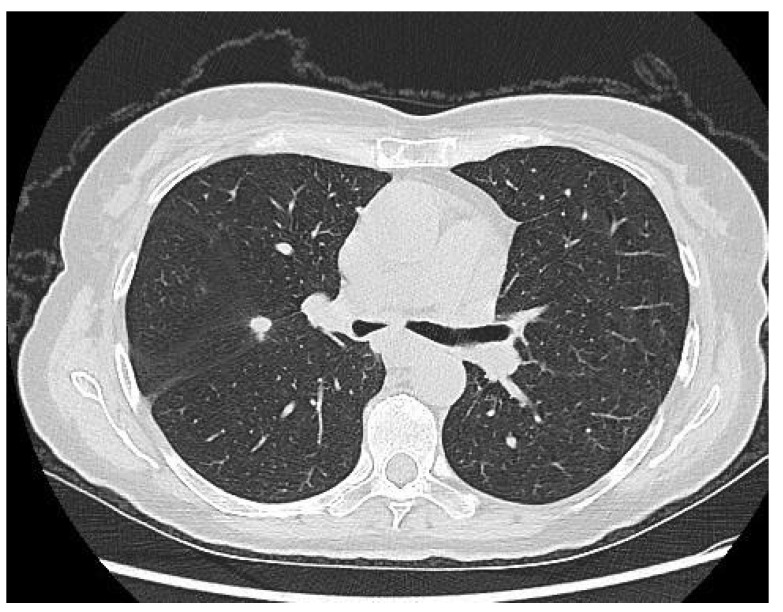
Computed tomography revealing extrinsic compression of the medial third of the esophagus in association with suspect pulmonary nodules.
